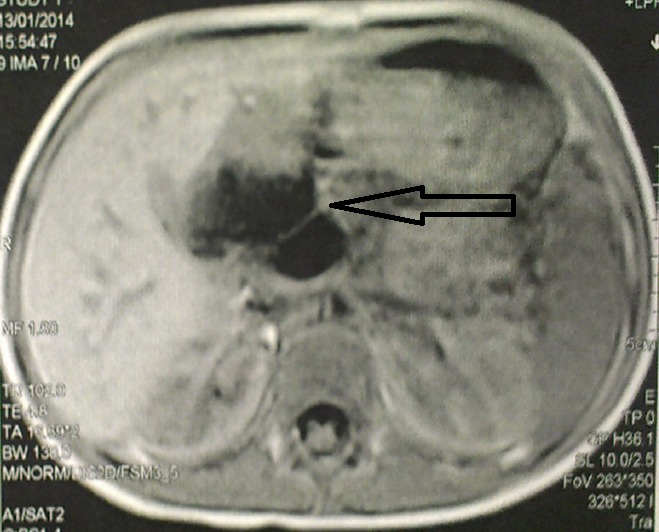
Abdominal MRI: cystic mass of the hepatic hilum in intimate contact with the duodenum, pulsing gallbladder and head of the pancreas
